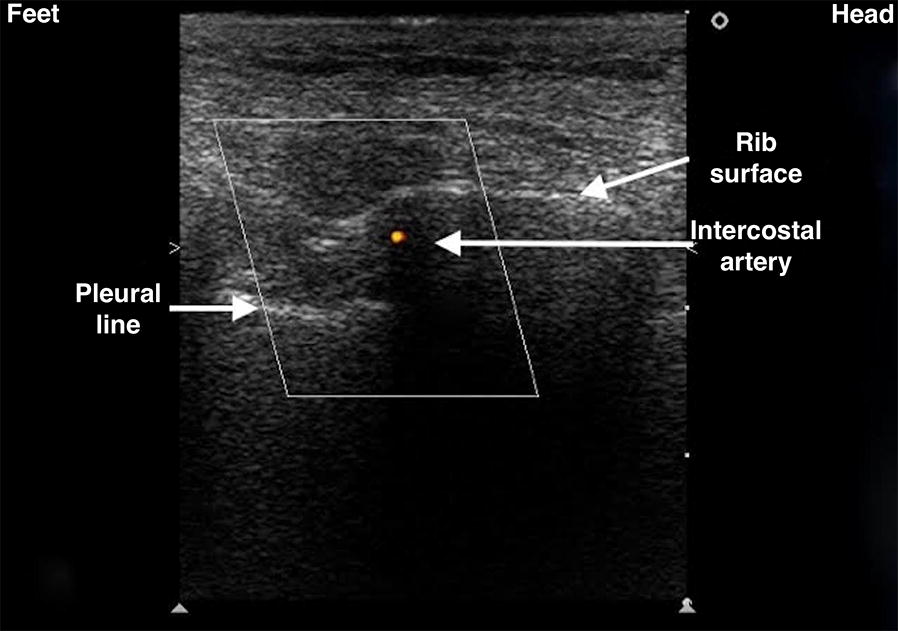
Visualisation of intercostal vessels using Doppler ultrasound. The probe marker is facing the patient’s head (on the right of the screen). The colour Doppler box should be placed on the bottom edge of the rib and the depth reduced until the rib does not occupy the entire screen. Pulse repetition frequency (PRF) should be reduced until pulsation is detected
